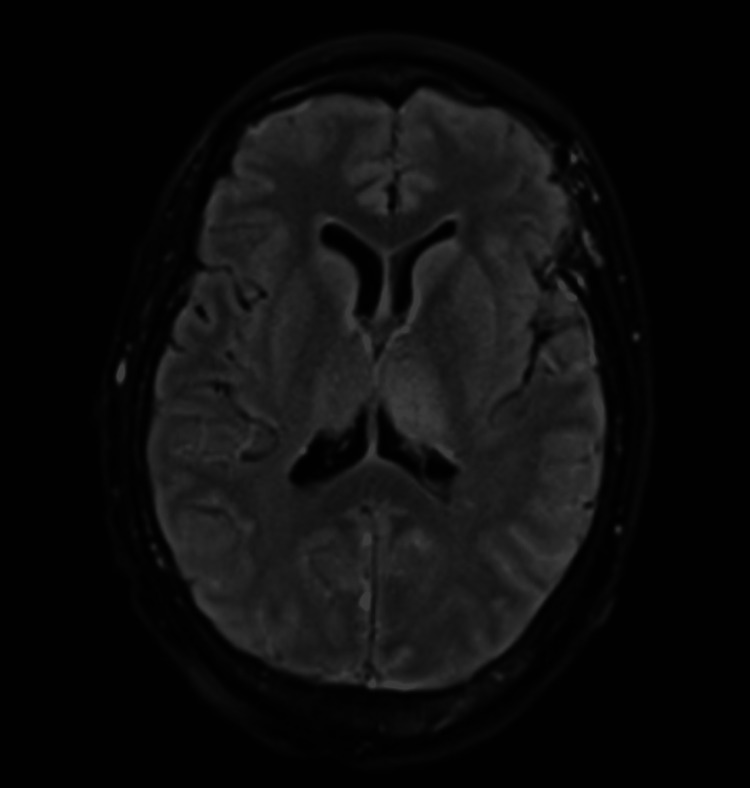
MRI of the brain FLAIR sequence in transverse view showing similar changes as noted on the DWI sequence indicating bilateral thalamic congestion. FLAIR: fluid-attenuated inversion recovery; DWI: diffusion-weighted imaging.
